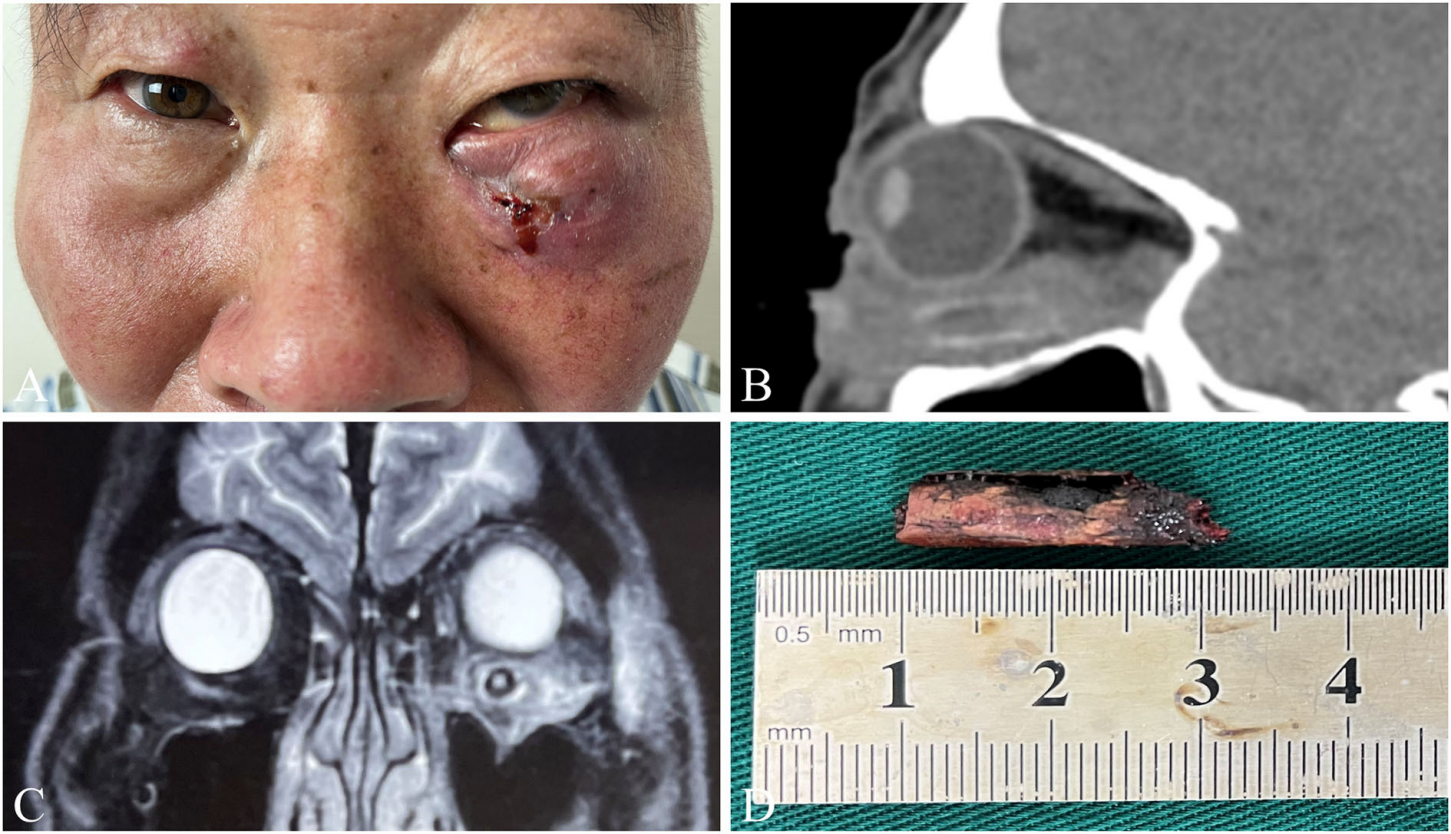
A representative case of IOWFBs. A 55-year-old man who suffered pain and swelling in his left eye after being injured by a branch and received two separate debridement operations to remove multiple branch fragments at a local hospital. A month after the second operation, he was transferred to our hospital for a thorough examination and treatment as his would worsened. (A) Since a fistula with mucinous secretions in the lower eyelid was exhibited, he was admitted to our hospital 1 month after the second operation. (B) The sagittal CT scan showed a high-density shadow under the left eyeball, with a size of about 20 × 6 mm. (C) MRI detected a hollow columnar shadow below the inferior rectus muscle of the left eye. (D) A branch ~20 mm long was taken out by exploratory surgery.
